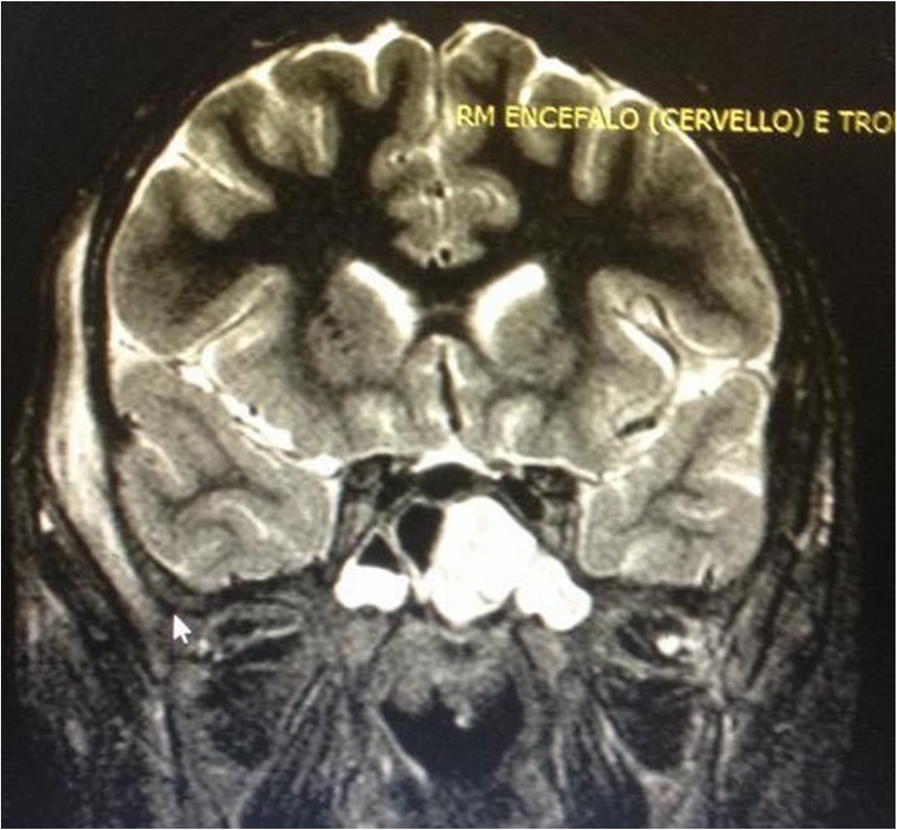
Preoperative MR imaging (coronal view): signs of bilateral sphenoiditis (left more than right)
